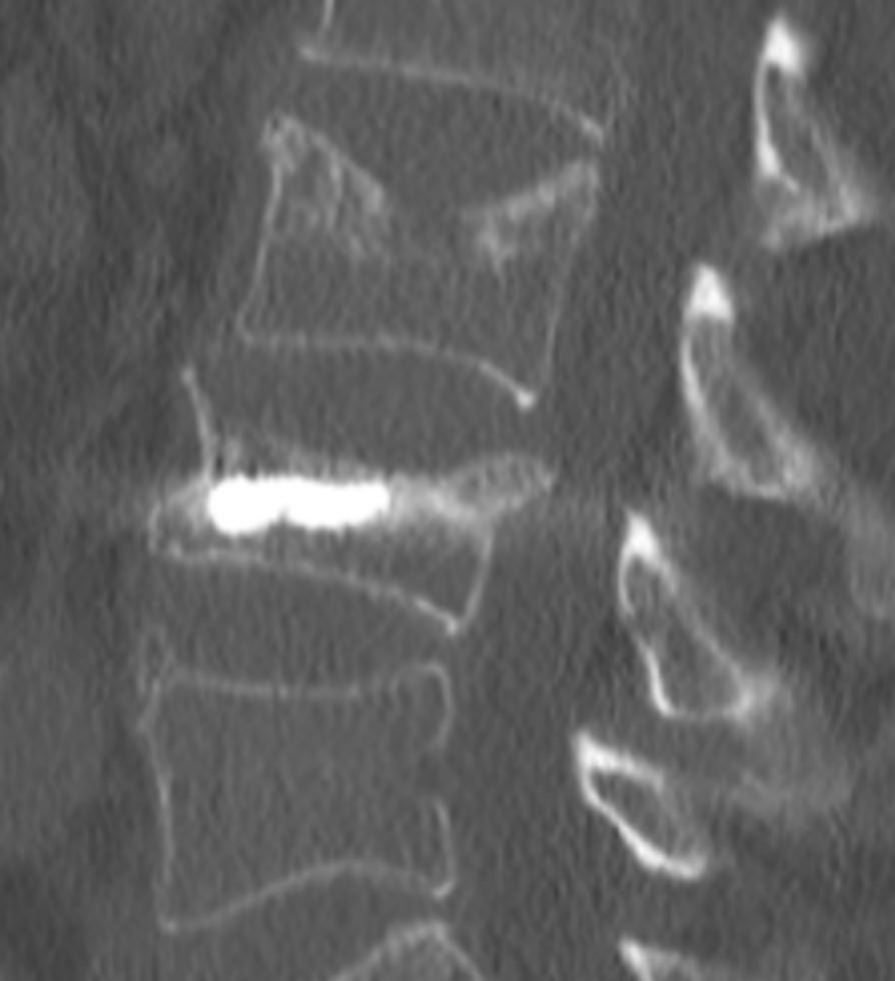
Sagittal CT-Scan showing an ASF after kyphoplasty in the superior vertebral body
